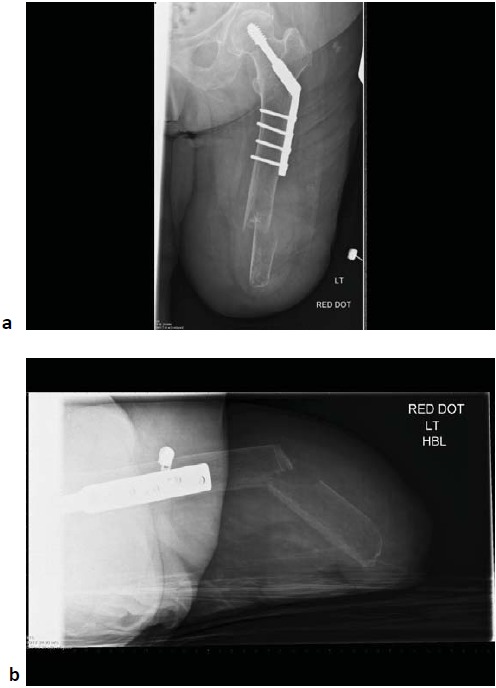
Antero-posterior (a) and lateral (b) radiograph on admission showing a distal femoral shaft fracture with a previous dynamic hip screw fixation.
